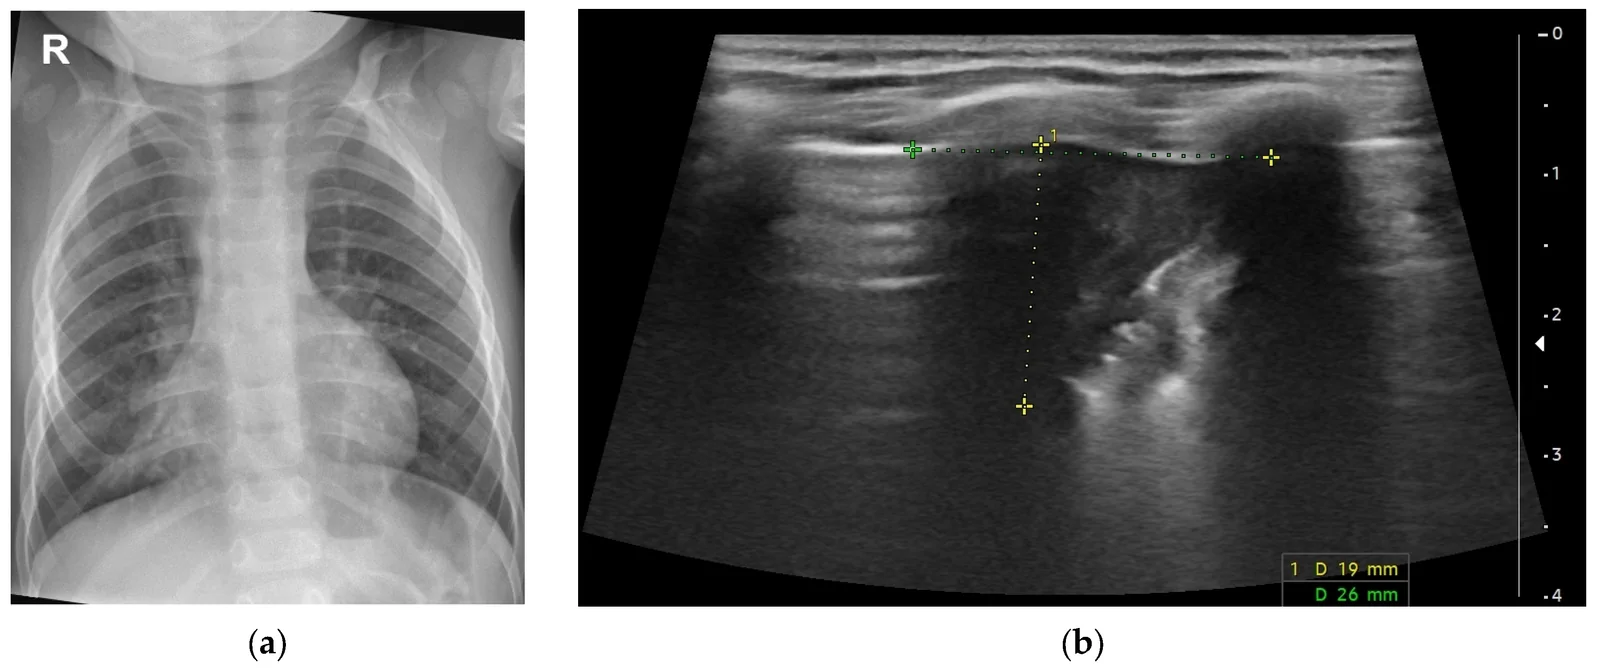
(a) Posteroanterior chest radiograph of a 1-year-old male with right middle lobe pneumonia. (b) Lung ultrasound of the same patient reveals pulmonary consolidation, pleural irregularity and an adjacent pleural effusion.
